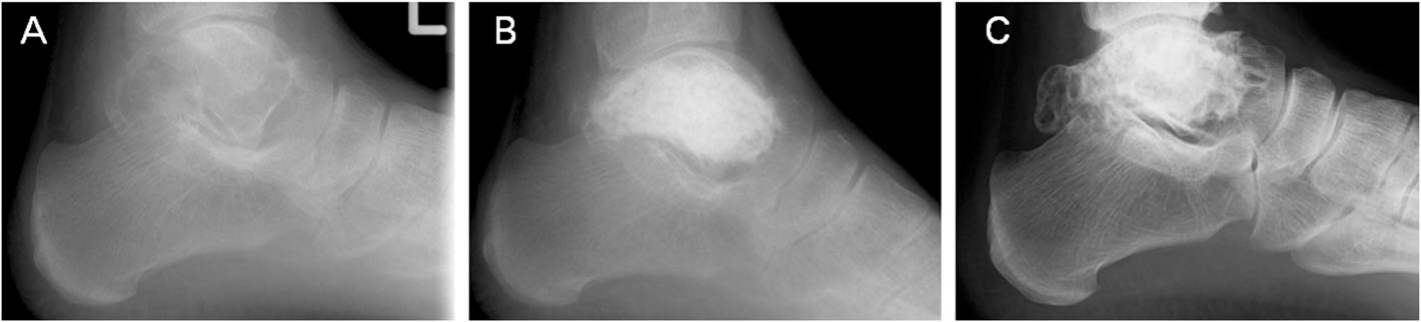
Chondroblastoma arising from the left talus. a Plain radiography upon referral showing a lytic lesion in the talus. b One month after surgery. c Fourteen years after surgery
